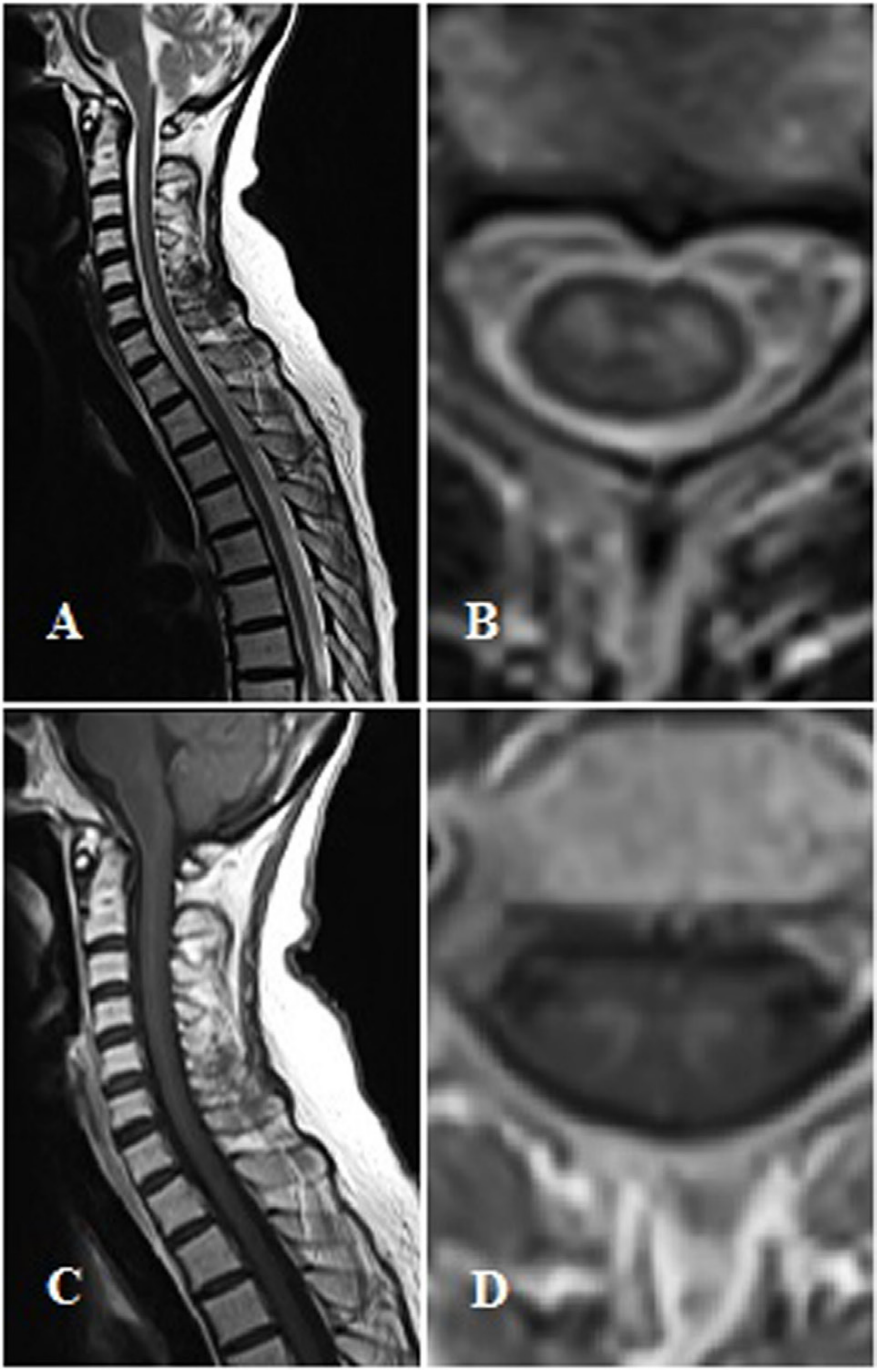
Magnetic resonance imaging findings. Panel (A,B) show T2-weighted sagittal and axial images. Panel (C,D) show T1-contrast sagittal and axial images.
